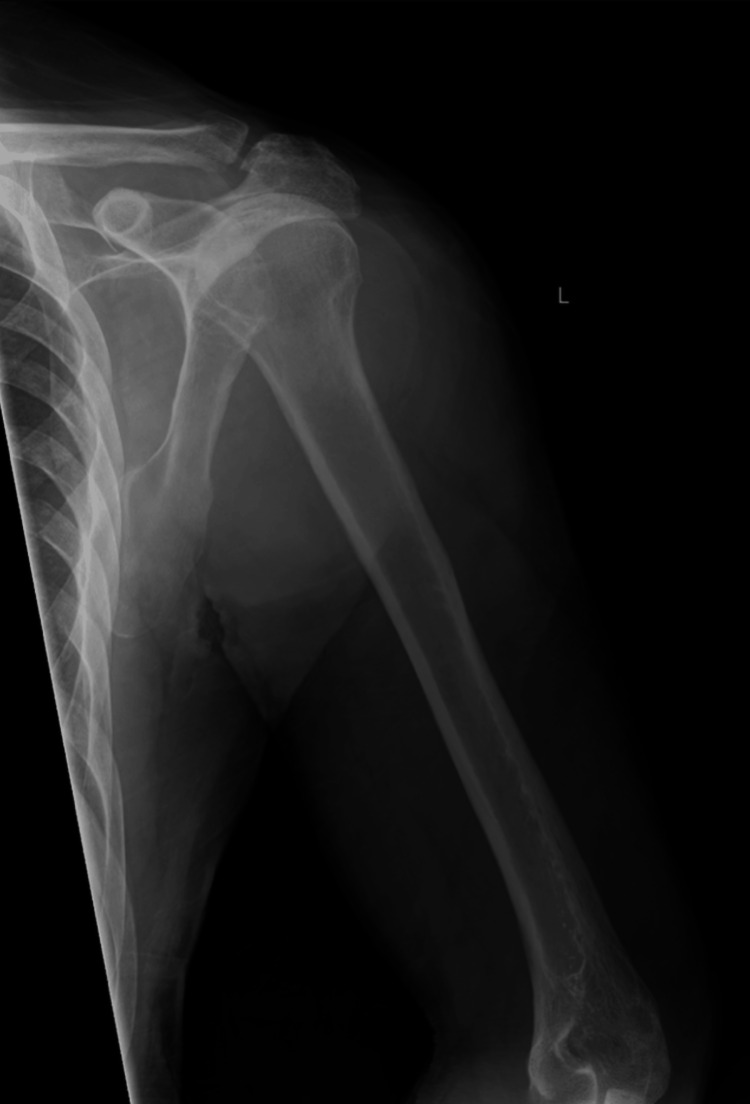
X-ray of the left shoulder and left upper arm Negative for any fractures or shoulder dislocation
